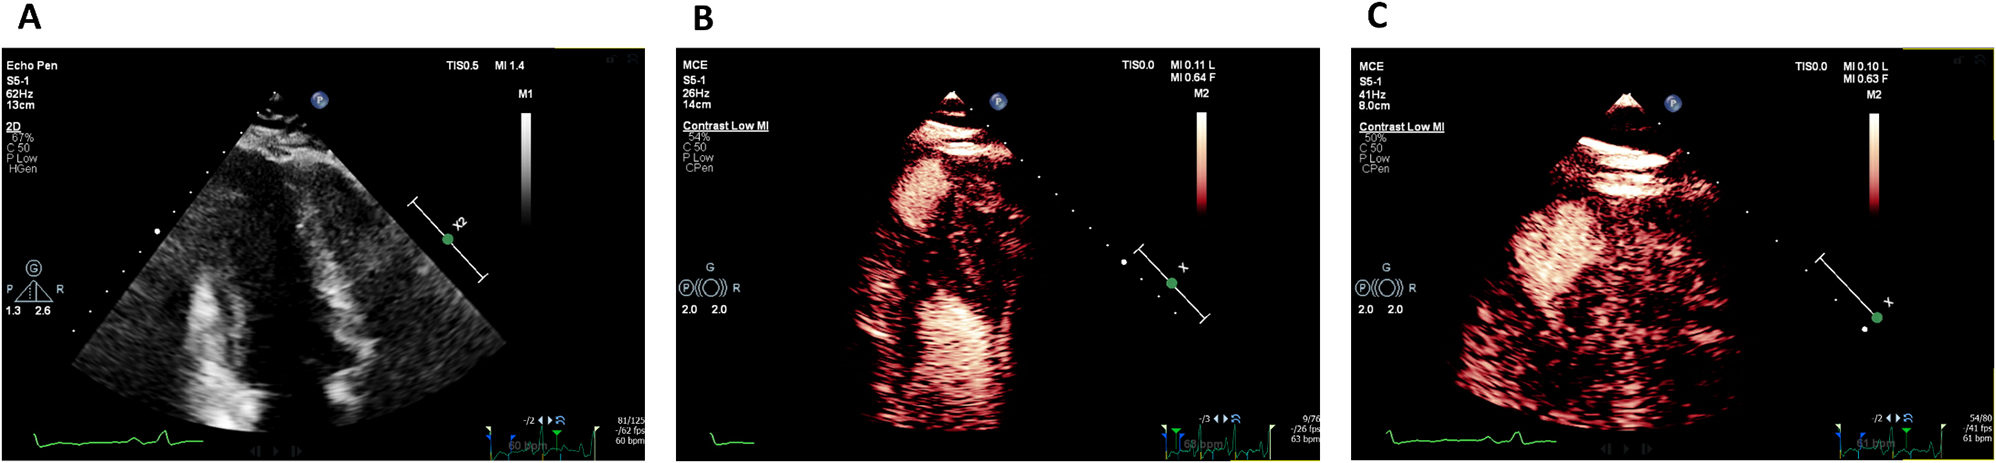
Unenhanced apical 3 chamber (A) vs contrast enhanced apical 3 chamber views (B & C) at end-systole in the same hypertrophic cardiomyopathy patient revealing a significant left ventricle apical aneurysm with no thrombus that was previously not visualised without the use of contrast enhanced echocardiography
